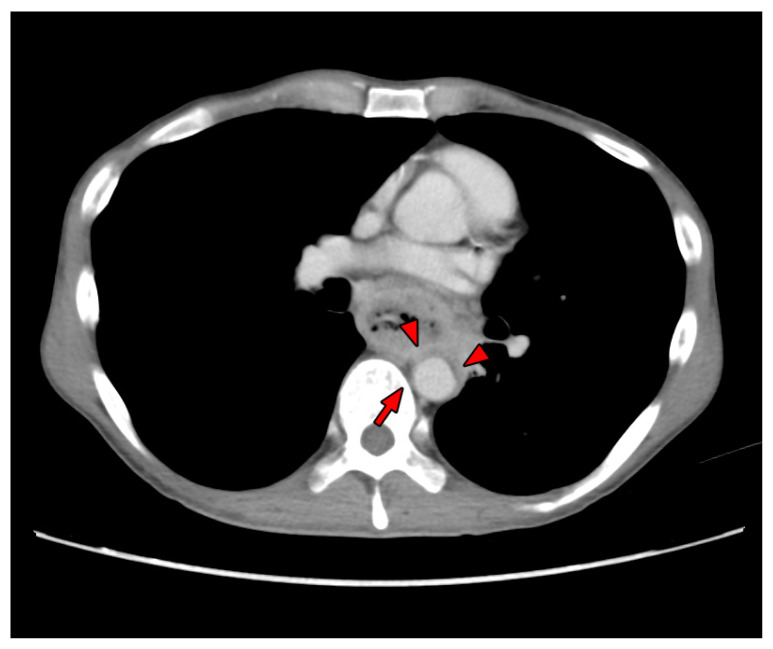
Computed tomography image of a patient with esophageal cancer before receiving elective thoracic endovascular aortic repair (TEVAR). The arrowheads are esophageal cancer invading the aortic wall. The arrow demonstrates the obliteration of the triangular fat space between the esophagus and thoracic aorta. These findings are indicated for elective TEVAR.
